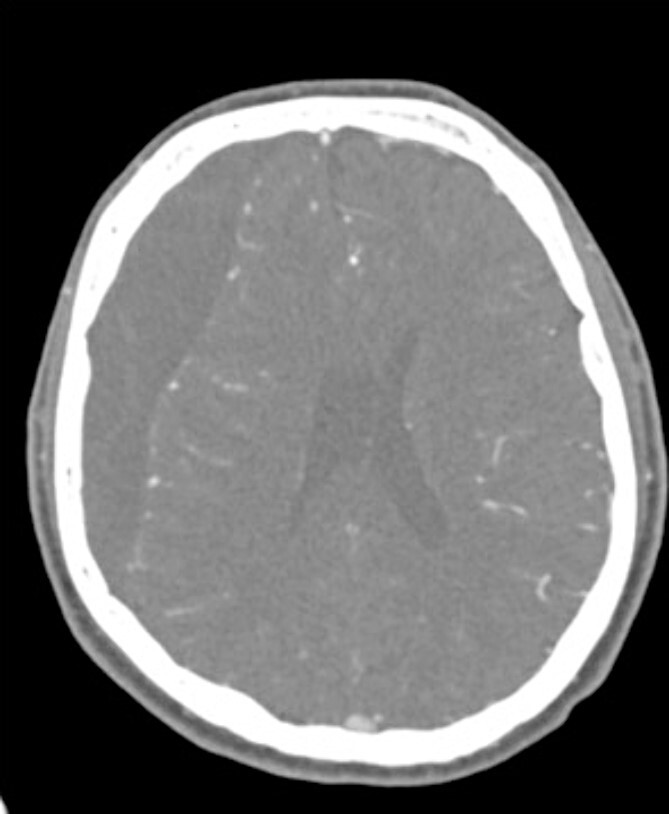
Preoperative CT imaging from Case 1.
